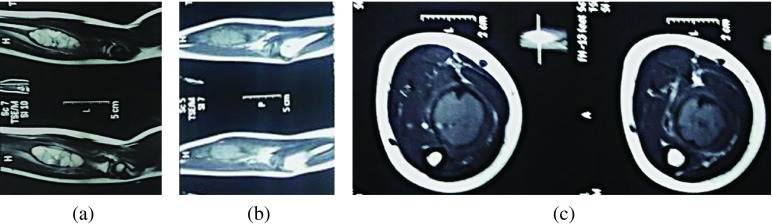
(a) T2 weighted sagittal MR images showing meta-diaphyseal expansile lesion with multiple hyperintense cysts with fluid-fluid levels and surrounding complete hypointense rim, (b) T1 weighted sagittal MR images showing iso to hypointense lesion, (c) T1 weighted sagittal MR images showing well marginated expansile iso to hypointense lesion.
